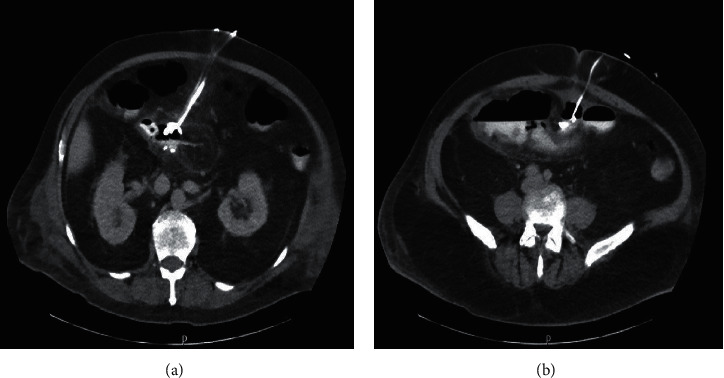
CT showing drainage catheters at the anastomotic site.
